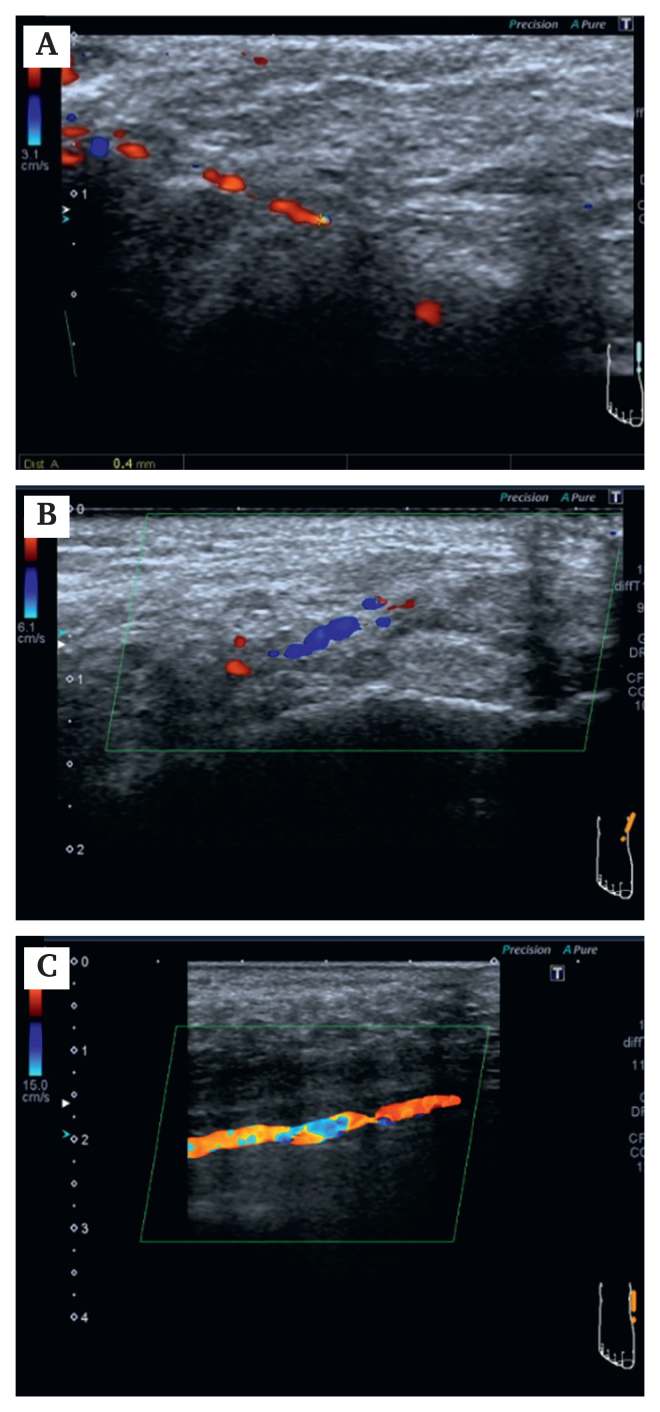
Sonographic images of the lateral plantar artery at (A) baseline, (B) after Rheocarna therapy, and (C) after pedal bypass surgery. Quantitative measurements are provided in Supplementary Table S1. (A) On admission, duplex ultrasound showed severely diminished flow in the lateral plantar artery with a luminal diameter of 0.4 mm. (B) Following low density lipoprotein apheresis therapy, gradual improvement in flow signals was observed, and the artery diameter increased to 0.8 mm after 2.5 months. (C) One month post-operatively, the diameter had further increased to 1.4 mm.
